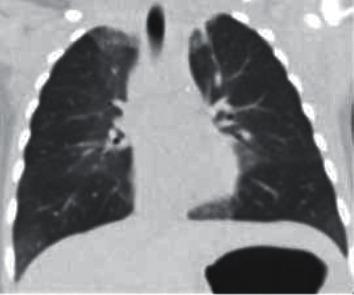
CT chest coronal view shows patchy central groundglass opacities and lower lobe atelectasis.
